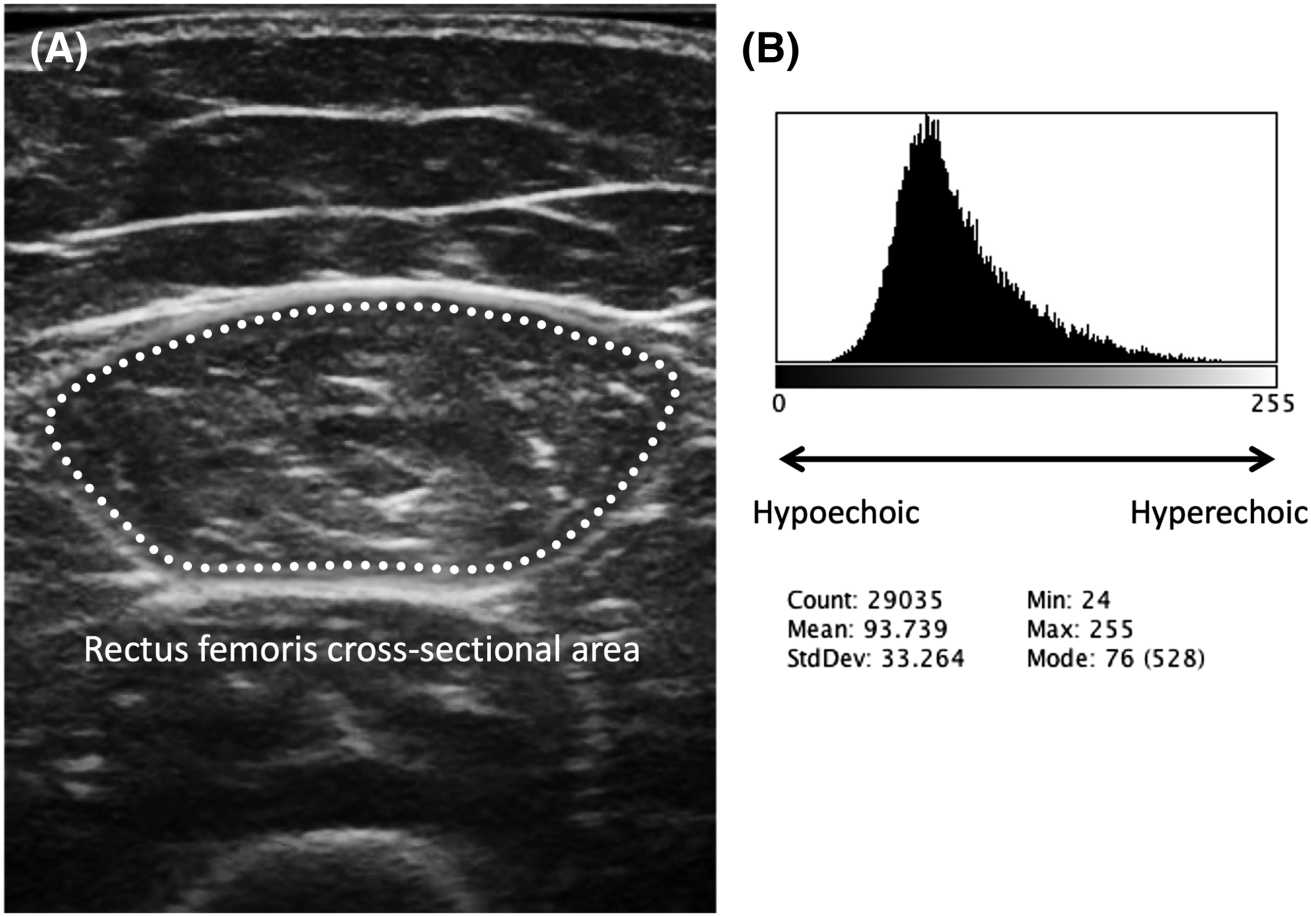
(A) Ultrasound measurement of the rectus femoris muscle cross‐sectional area (RF‐CSA). (B) Histogram of 8‐bit gray‐scale analysis of rectus femoris muscle echo‐intensity (RF‐EI). The region of interest was selected within the outline of the rectus femoris to include as much of the muscle as possible without any surrounding fascia.
